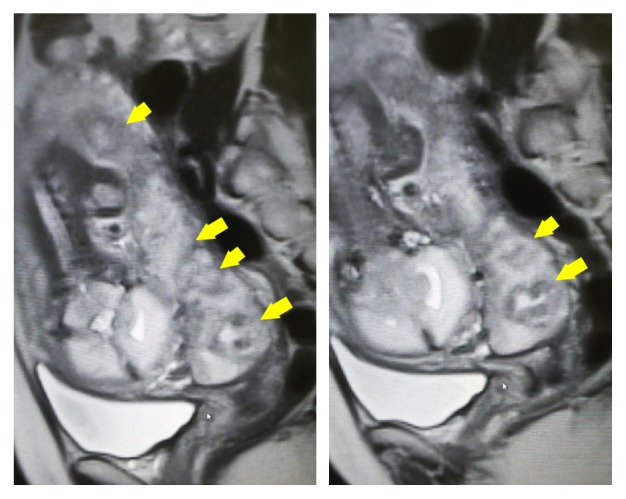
T2-weighted image showed a mixture of hyperintense and hypointense lesions (arrows) in the placenta, representing cotyledon infarction.
